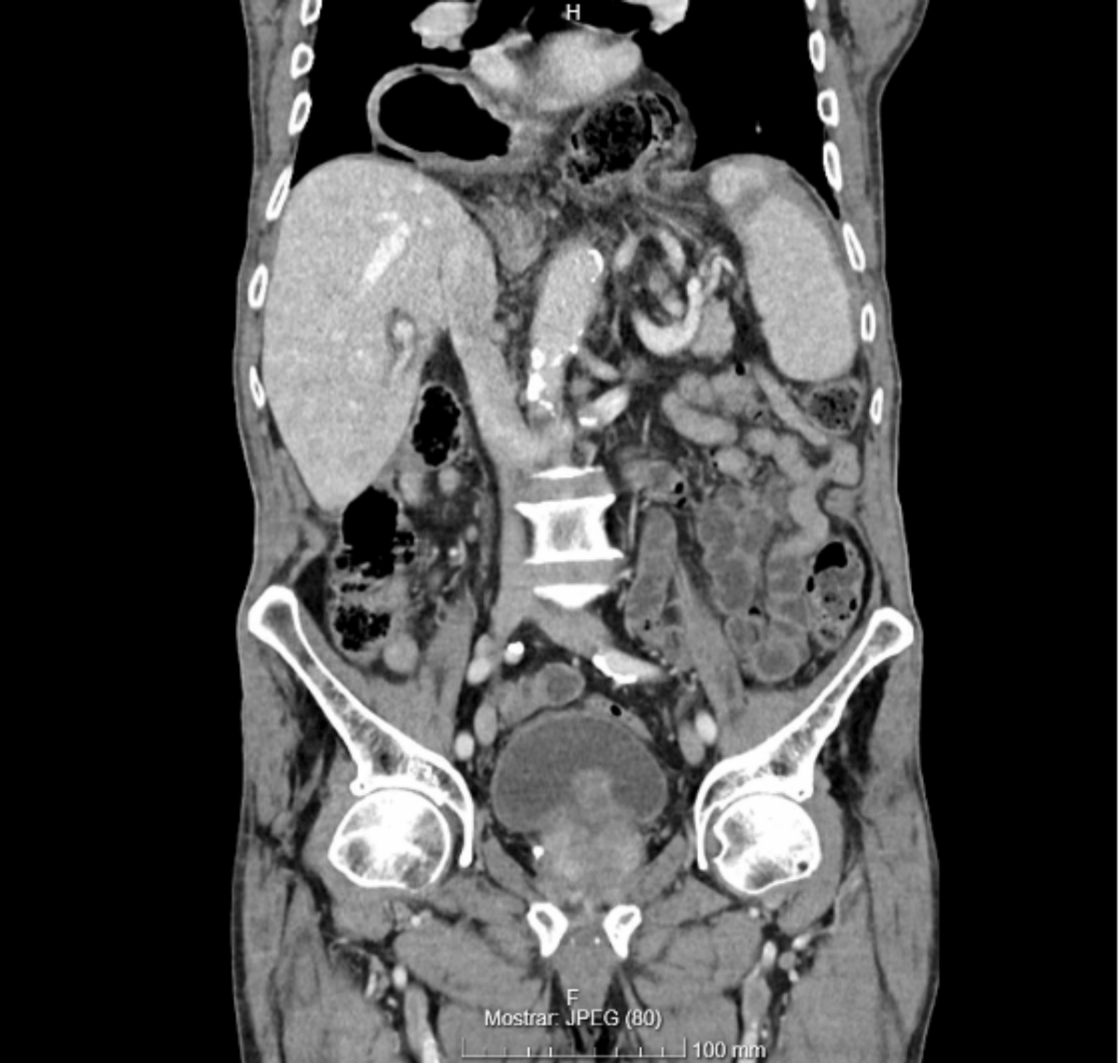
Coronal CT scan showing the large hiatal hernia
